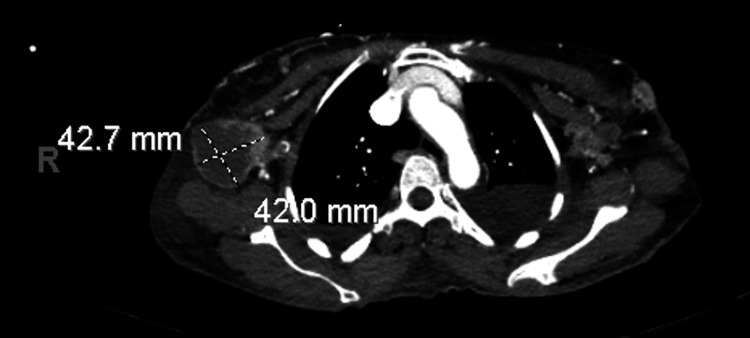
CT scan of the chest with contrast showing a hypovascular mass measuring 42.7 × 42 mm with thickened enhancing walls located within the right axilla, raising concerns for an enlarged, necrotic lymph node
